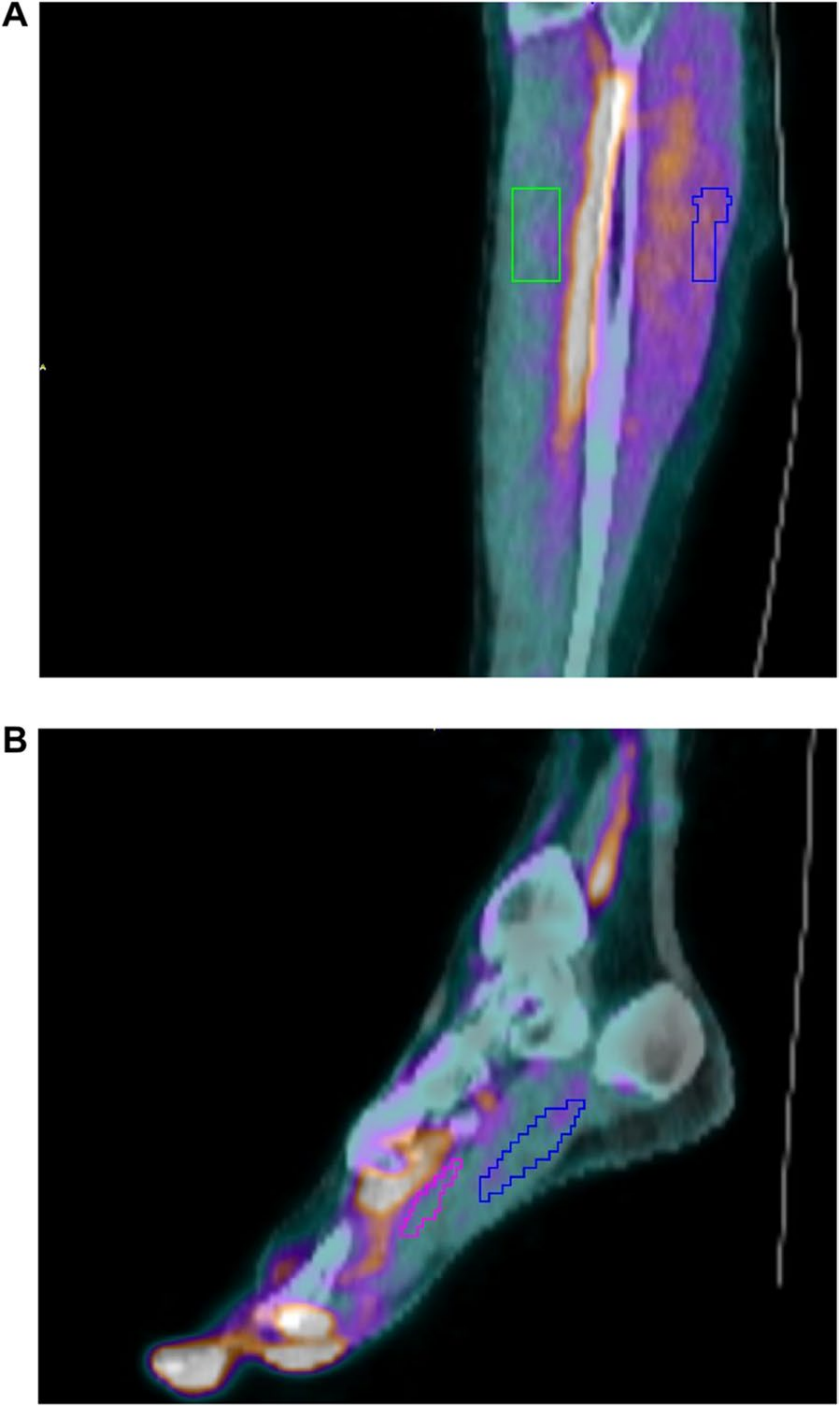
Example of parametric images of tissue perfusion (sagittal view) overlayed on CT. A Right lower leg with VOIs for GAS (green) and TA (blue). Color scale ranges from 0 to 6 mL/min/100mL. B Right foot with VOIs for FDB (pink) and AH (blue). Color scale ranges from 0 to 4 mL/min/100mL. Since the ARG method does not include blood volume correction, blood vessels and tissue with high blood volume appear as high perfusion
